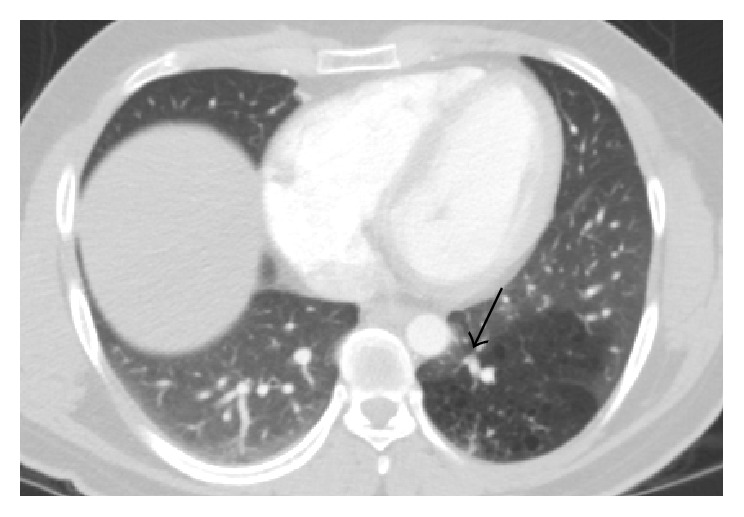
CT angiography shows dedicated pulmonary artery and vein supplying the hypolucent area. Small cysts can be appreciated within the hypolucent area.
